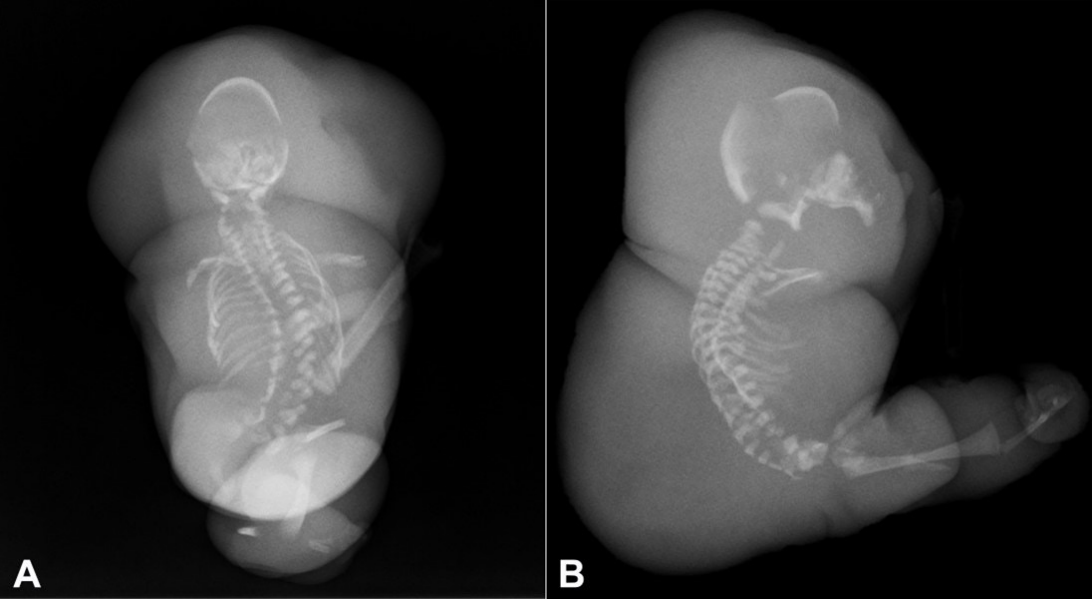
Postmortem Xray. A - AP view; B - lateral view demonstrating hypoplasia of the appendicular skeleton, small, malformed cranium, and thoracolumbar scoliosis with multiple vertebral anomalies.
